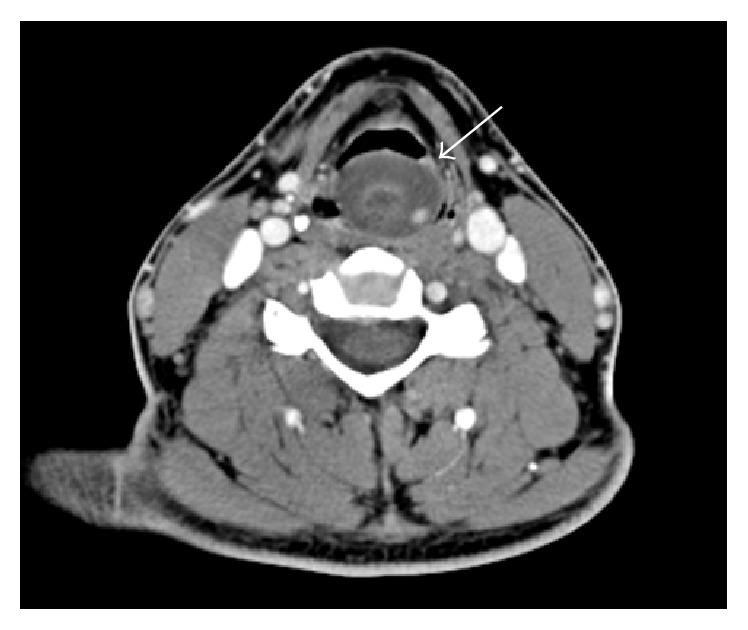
Contrast-enhanced CT scan of the neck. A well circumscribed, focally enhancing mass is noted in the left pyriform sinus (arrow).
